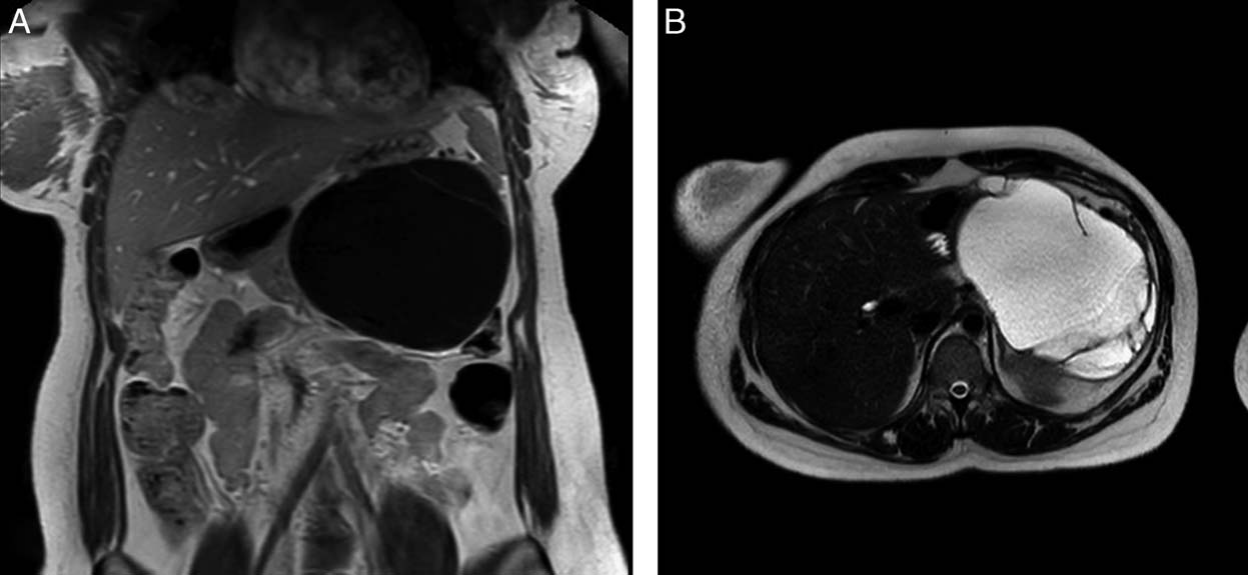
The 18×16 cm mucinous cystadenoma at the preoperative contrast-enhanced magnetic resonance imaging. A, Coronal section in T1 weighted. B, Transverse section in T2 weighted.
